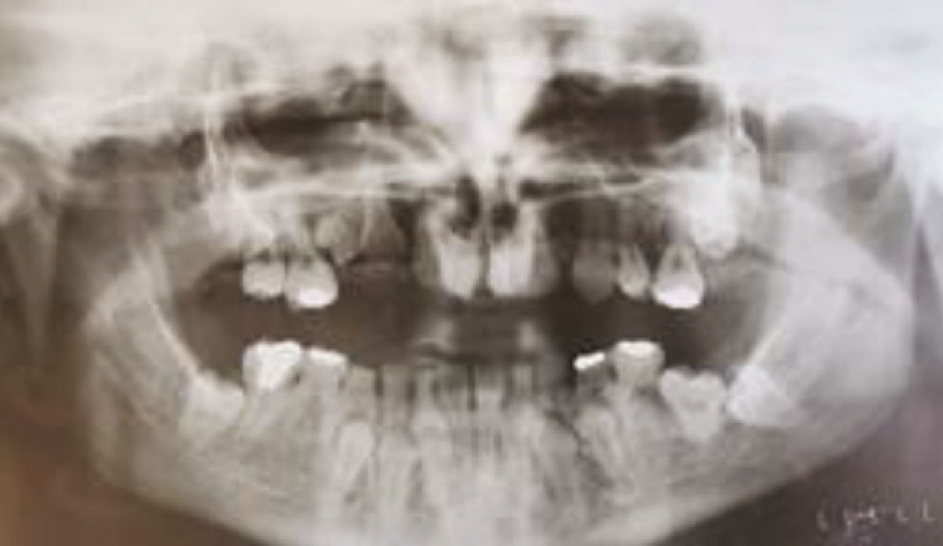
Panoramic radiograph taken at age of 16 before orthodontic treatment.
